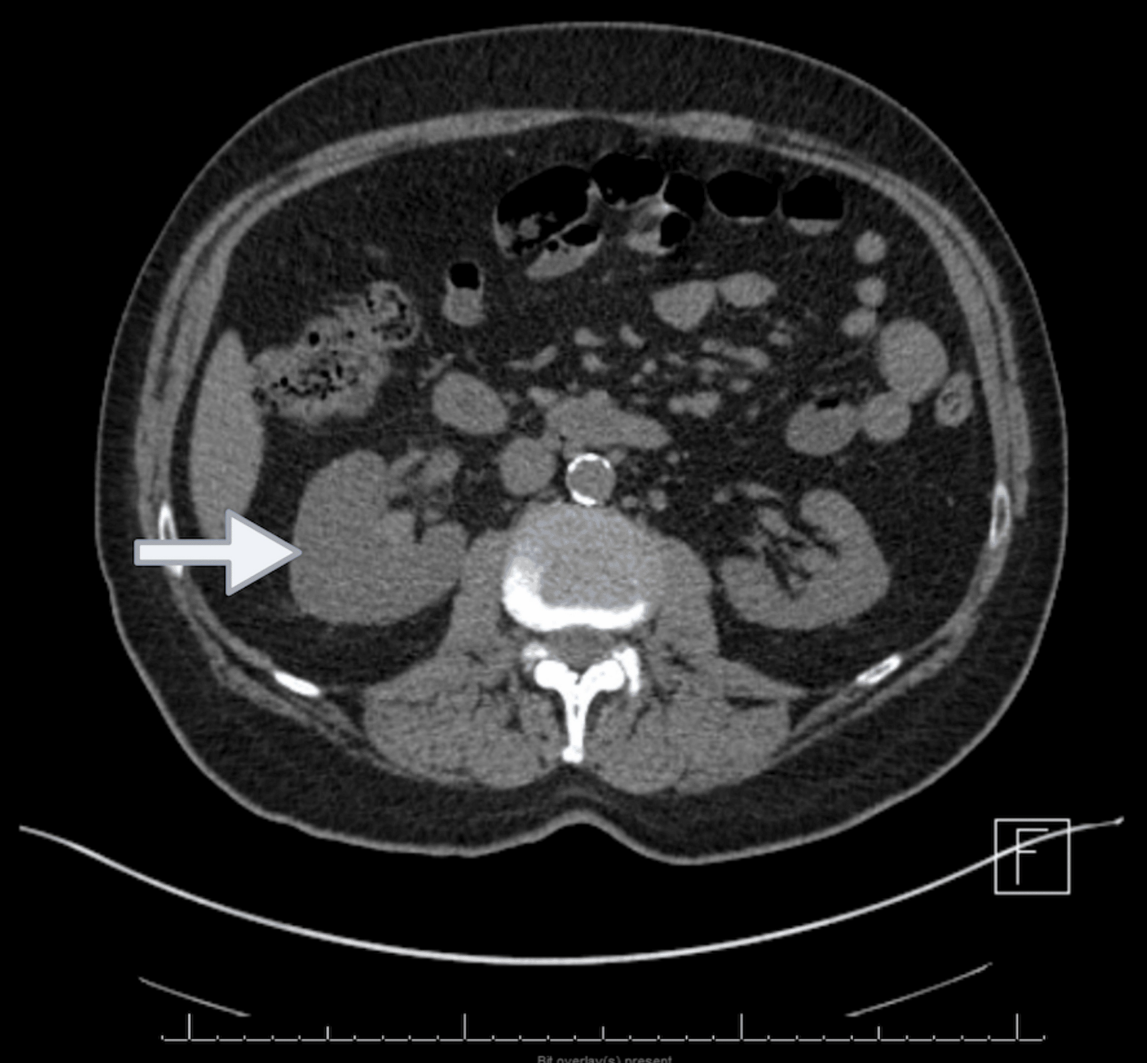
CT abdomen/pelvis showing initial right renal hemorrhage with right hydronephrosis (arrow)
